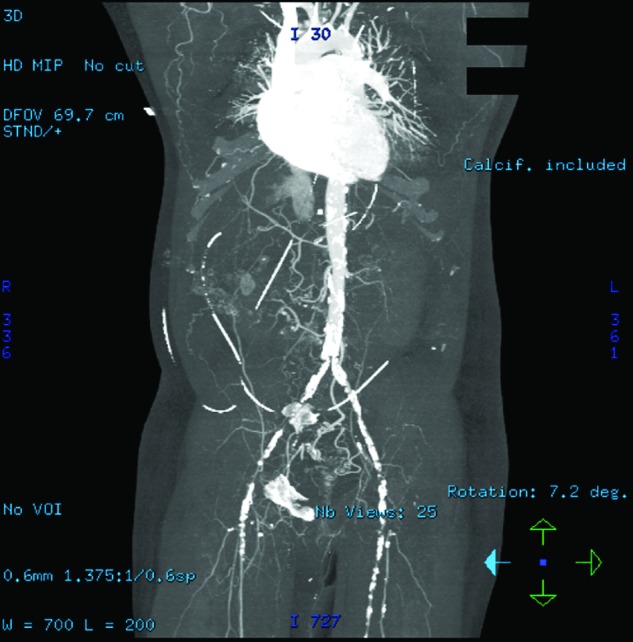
Three-dimensional computed tomography angiogram reconstruction demonstrating extensive severe luminal narrowing of the infrarenal abdominal aorta, bilateral common iliac, external iliac, and common femoral arteries by calcified and noncalcified plaques.
